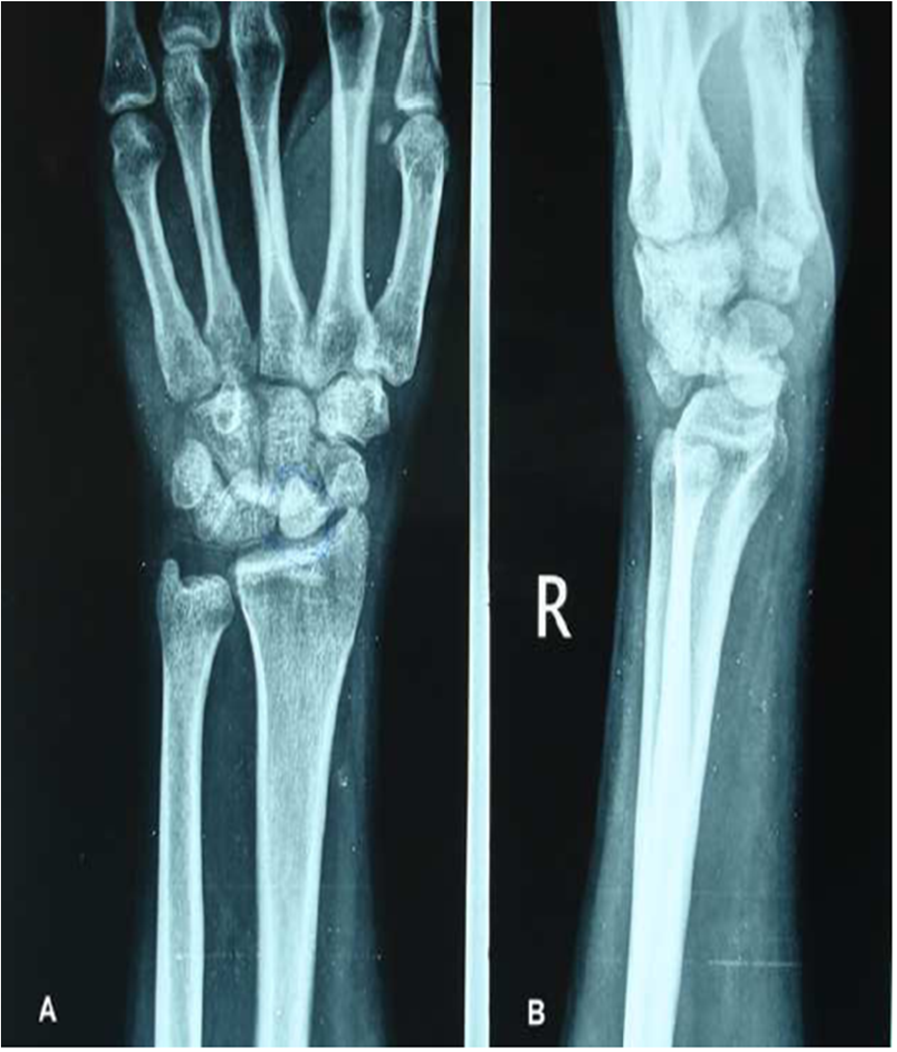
Preoperative radiograph showing a neglected transscaphoid perilunate dislocation; (A) Anteroposterior and (B) Lateral views.
